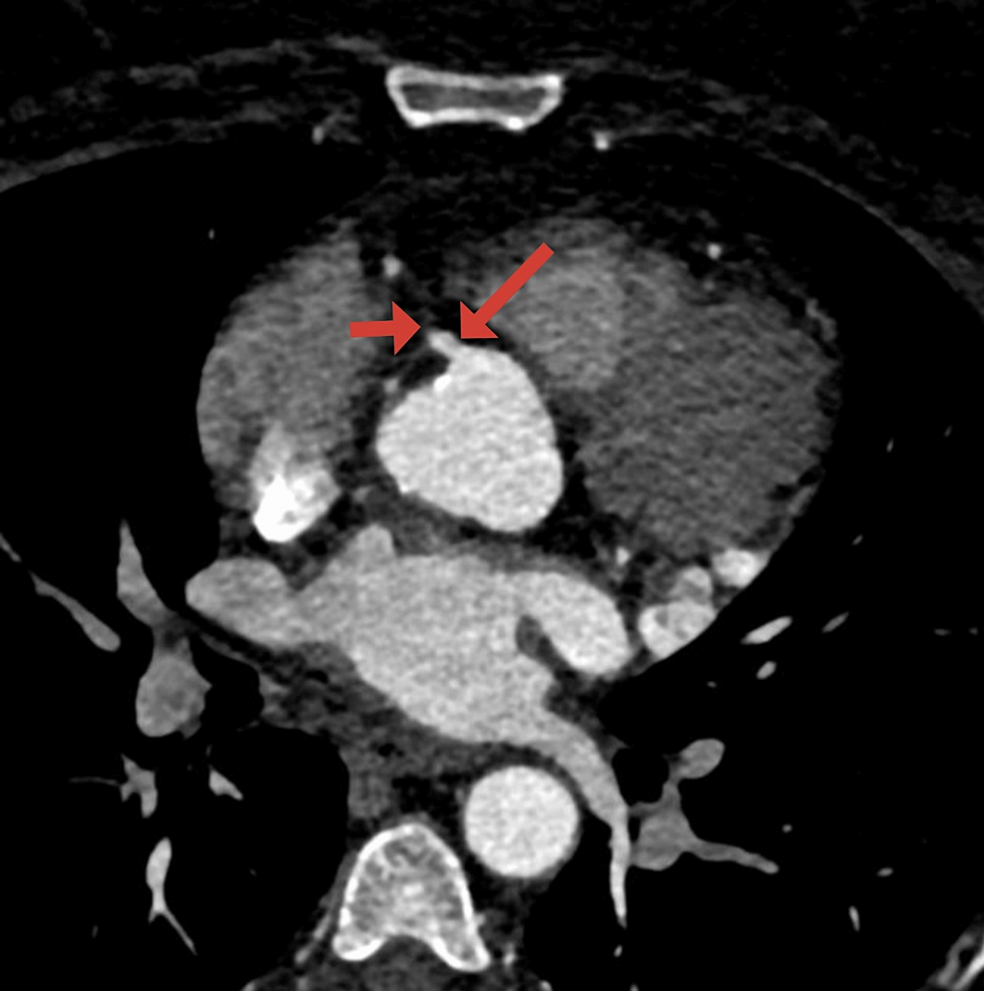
Coronary computed tomography angiography axial view of the heart. Axial view of the heart demonstrating contrast-enhanced proximal segment of the right coronary artery (long arrow) with an abrupt decrease in contrast flow in the mid-segments of the vessel (short arrow). There is no evidence of pericardial effusion.
